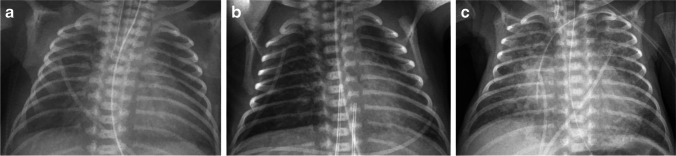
Evolving lung appearance on radiography in a premature newborn. a Anteroposterior (AP) chest radiograph in a 1-day-old boy born at 24 weeks of gestation shows diffuse, fine granular opacities throughout the lungs, often with air bronchograms. b AP chest radiograph of the same boy at 2 days old. Surfactant had been administered and the boy was extubated. At this time the lungs appear clear and better inflated. c AP chest radiograph in the same boy at 4 days of age shows that his lungs have become much more opaque and coarse, with mixed lucent and opaque areas resulting in reintubation
